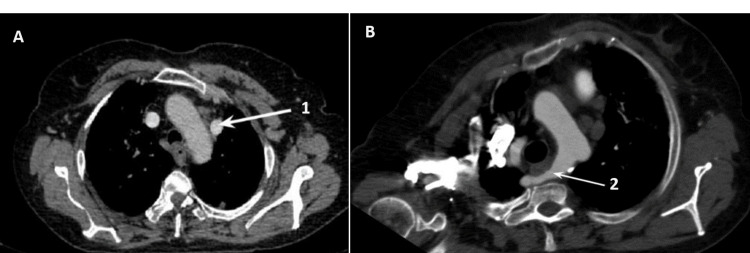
CT scan (A) Left superior vena cava (1). (B) Aberrant right subclavian artery (2).
